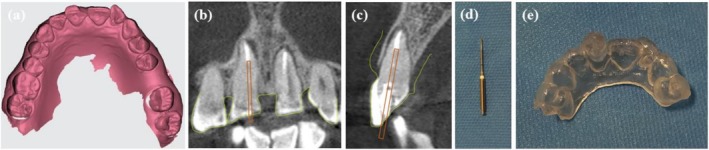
(a) Intraoral scan of the maxillary arch using STL format. (b, c) Virtual planning of coronal and sagittal plane of the guide drill placement to remove the fiber post. The drill was positioned along the long axis of the fiber post set up to its apical tip. (d) Munce discovery bur #2. (e) Endodontic guide. The STL file and the DICOM file obtained by CBCT were virtually merged, and a guide was fabricated using a 3D printer.
